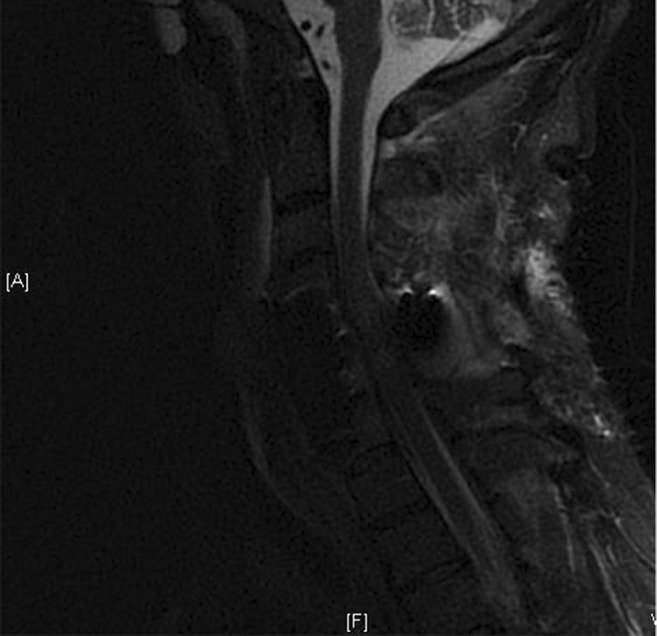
Sagittal MRI view status post‐anterior cervical discectomy and fusion and posterior spinal fusion hardware placement for epidural abscess, osteomyelitis, and diskitis with interval improvement of epidural abscess and cord compression.
